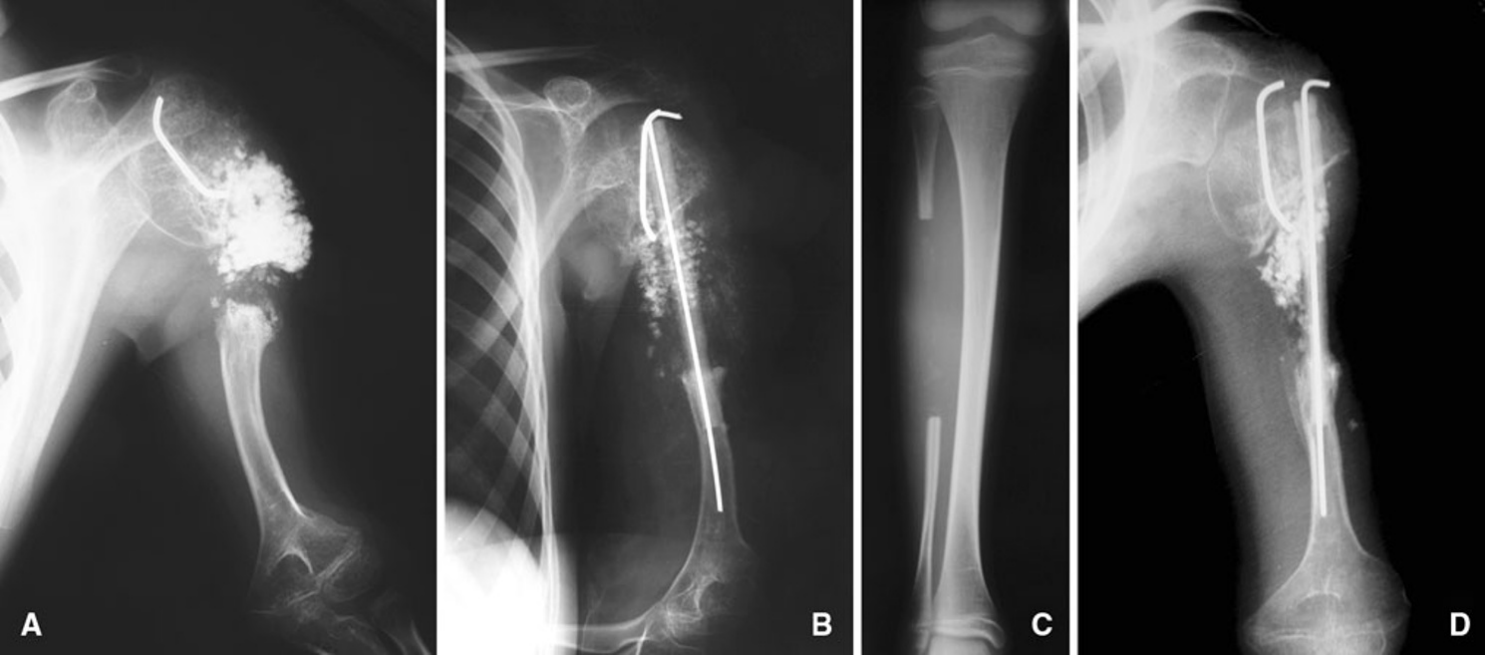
Anteroposterior radiograph (a) shows proximal humerus nonunion with a large defect containing allografts. After the operation at author’s institution, plain radiograph (b) shows reconstructed defective area with fibular autograft that was secured by one *K* wire. Fibular autograft was obtained from the contralateral extremity (c). At the third-month follow-up, plain radiograph shows graft incorporation in the distal part of the fibular graft with inadequate healing of the proximal part (d)
